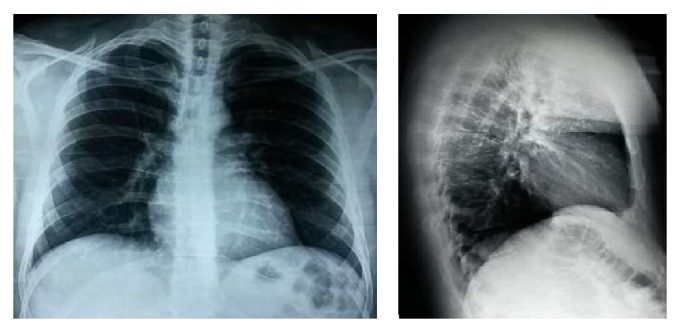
Posteroanterior and lateral chest X-rays of the patient.
